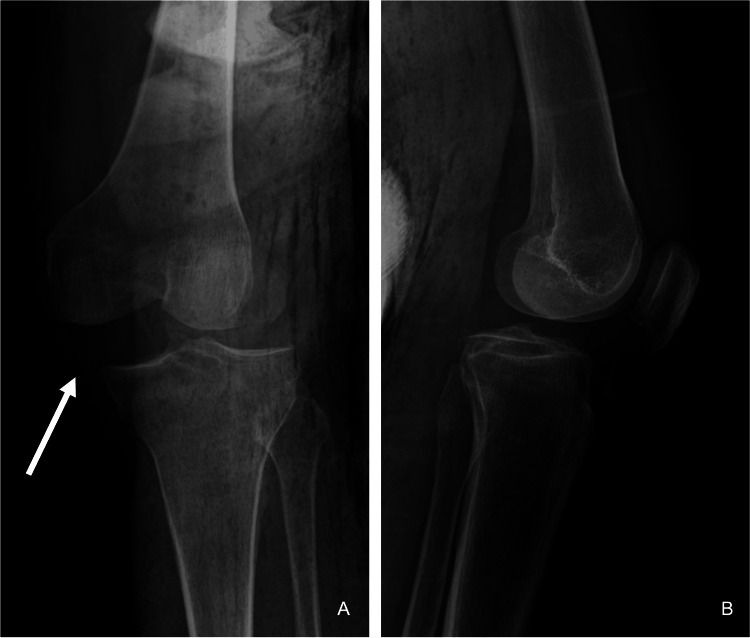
Pre-reduction radiographs of postero-lateral knee dislocation A: anteroposterior; B: lateral radiographs of the left knee showing postero-lateral dislocation with lateral displacement and rotational malalignment of the tibia relative to the femur (marked by a white arrow).
